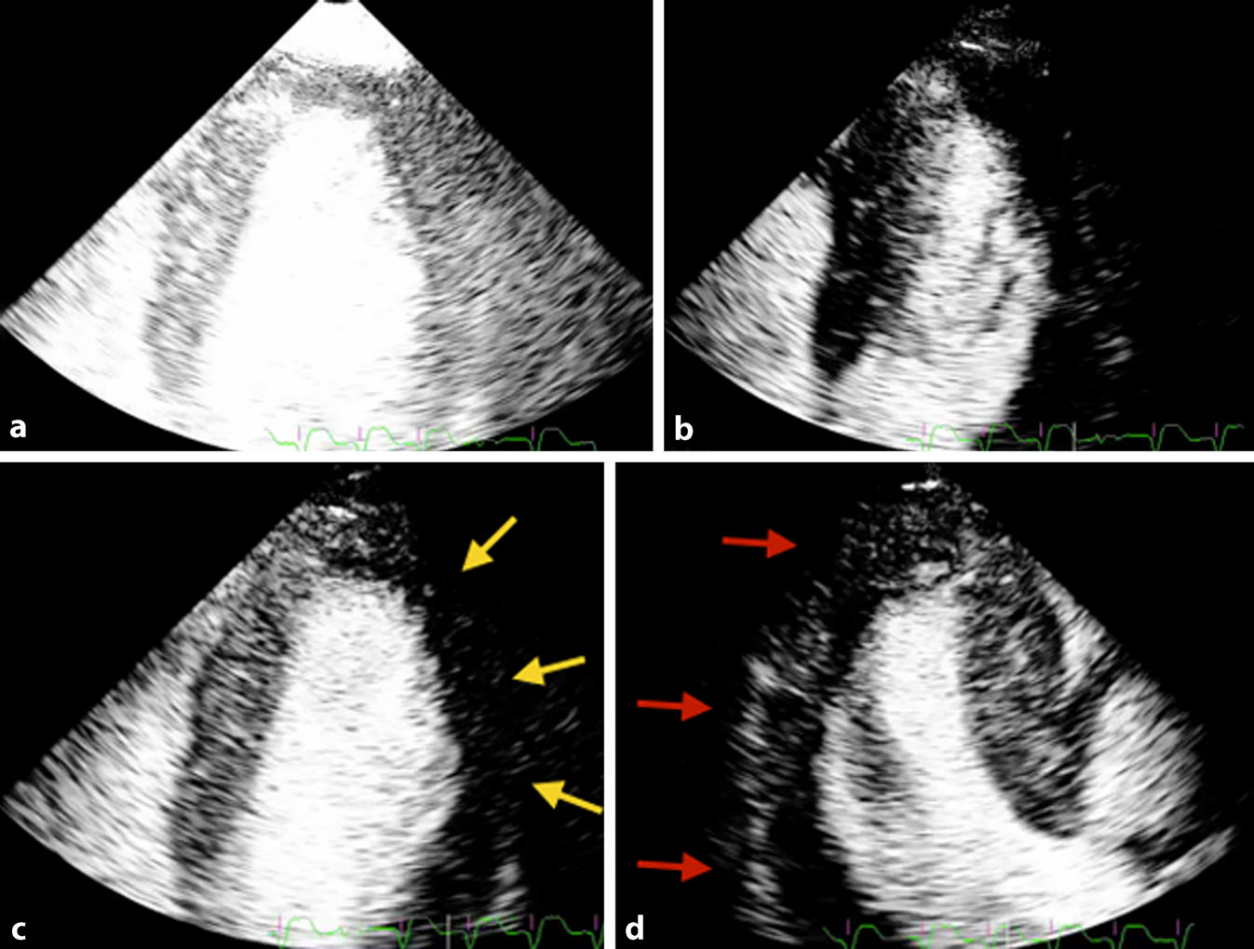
Real-time low-MI myocardial contrast echocardiography. a, b Immediately after flash function; c as contrast appears in the myocardium, *yellow arrows* show a large area of perfusion defect in the lateral wall in the apical four-chamber view; d *red arrows* show the perfusion defect in the inferolateral wall in the apical three-chamber view. Coronary angiography findings were consistent with a critical stenosis in the left circumflex artery
